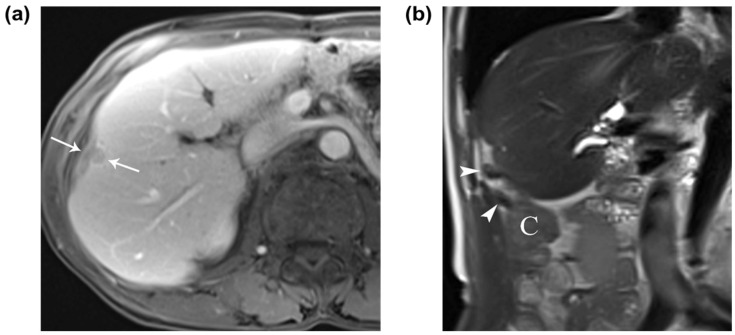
A 69-year-old man was admitted to our hospital for the evaluation and treatment of a hepatic mass. He had a history of pylorus-preserving pancreaticoduodenectomy and adjuvant chemotherapy for cholangiocarcinoma in the distal common bile duct 11 years previously and had developed liver cirrhosis due to alcohol abuse. Upon admission, laboratory findings were unremarkable. Contrast-enhanced computed tomography (CT) and gadoxetic-acid-enhanced magnetic resonance imaging (MRI) were performed to evaluate the hepatic mass. The MRI showed a 1.2 cm subcapsular hepatic nodule with typical findings of HCC in segment 5 of the liver, located adjacent to the cecum and ascending colon (Figure 1). Although the hepatic nodule has typical imaging findings of HCC, the possibility of hepatic metastasis cannot be ruled out. Thus, we decided to perform a percutaneous ultrasound (US)-guided core needle biopsy to distinguish between hepatic metastasis and HCC. RFA was selected as the treatment of choice after the nodule was confirmed to be HCC. RFA was performed under intravenous and local anesthesia. Before electrode placement, hydrodissection using 1 L of 5% dextrose solution was introduced under US guidance to minimize thermal injury to the adjacent colon. Tumor ablation was performed for 8 min after the placement of electrodes. A 1.2 cm subcapsular hepatocellular carcinoma in segment 5 of the liver in a 69-year-old man. (a) Contrast-enhanced axial T1-weighted MR image shows the washout of a 1.2 cm subcapsular hepatocellular carcinoma (arrows) on the portal venous phase. (b) A coronal T2-weighted MR image shows the perihepatic location of the appendix (arrowheads) and cecum (C) around the hepatocellular carcinoma.
